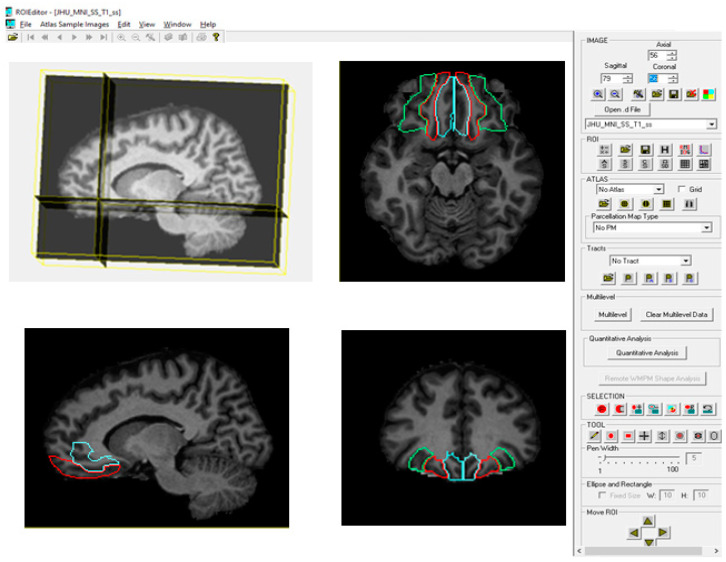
Identification of the gyrus rectus (blue), medial orbitofrontal gyrus (red), and lateral orbitofrontal gyrus (green) on normalized MRI images using ROI Editor.
